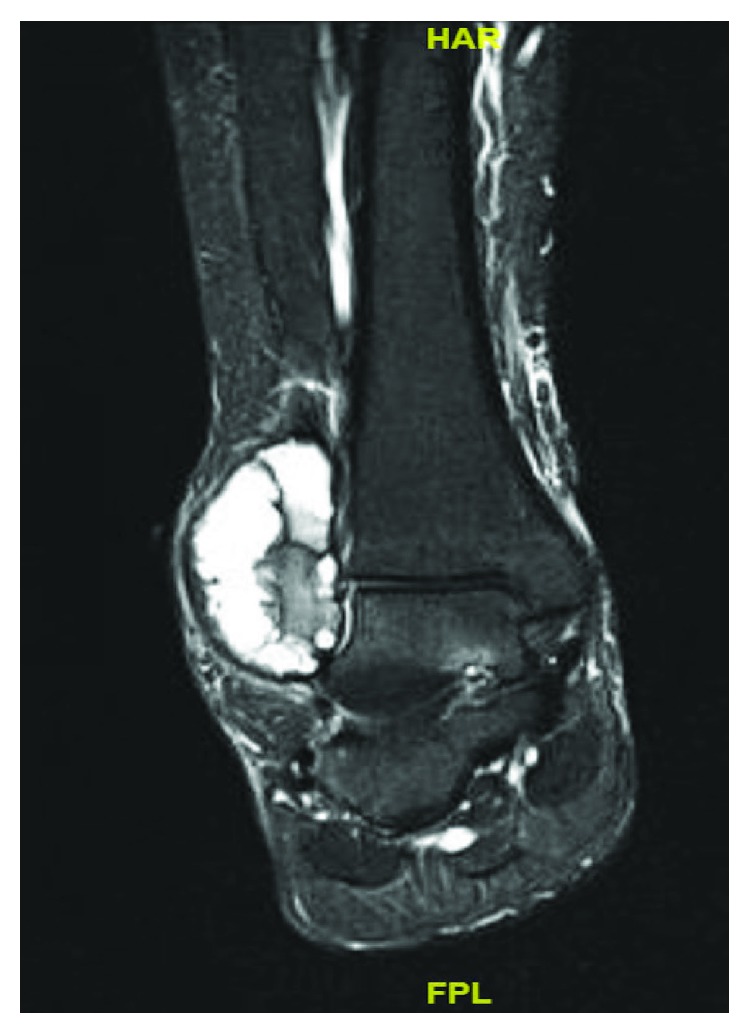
STIR (short tau inversion recovery) weighted MRI showing a homogeneously lobulated, hyperintense lesion with multiple fluid-fluid levels.
